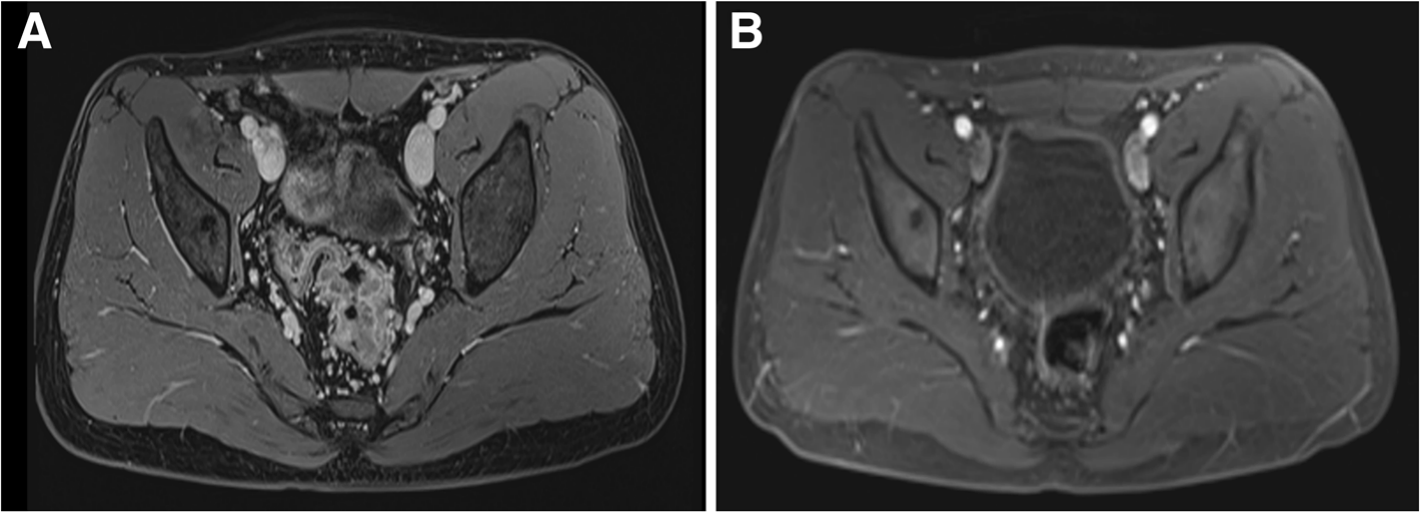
Magnetic resonance imagines (MRI) of the pelvis (a): Sagittal MRI of the pelvis showed rectal mass with involvement of perirectal fat and enlarged lymph nodes. b: Following 4 cycles of FOLFOX neoadjuvant chemotherapy, the follow up MRI reveals significant tumor regression, indicating response to treatment
